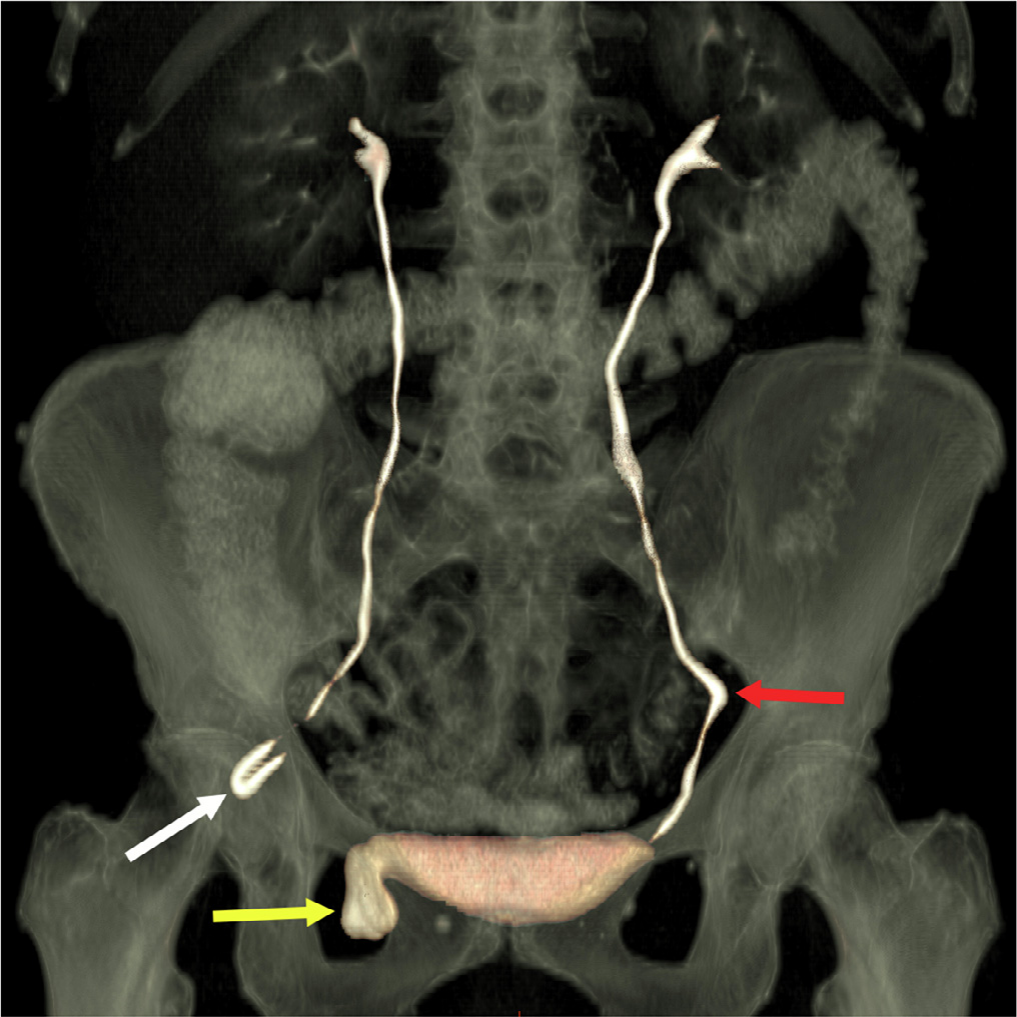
Coronal CT 3-D reconstruction with volume rendering demonstrating a right ureterosciatic hernia (white arrow), right bladder hernia (yellow arrow), and left ureteral deviation without herniation (red arrow) (color version of figure is available online).
